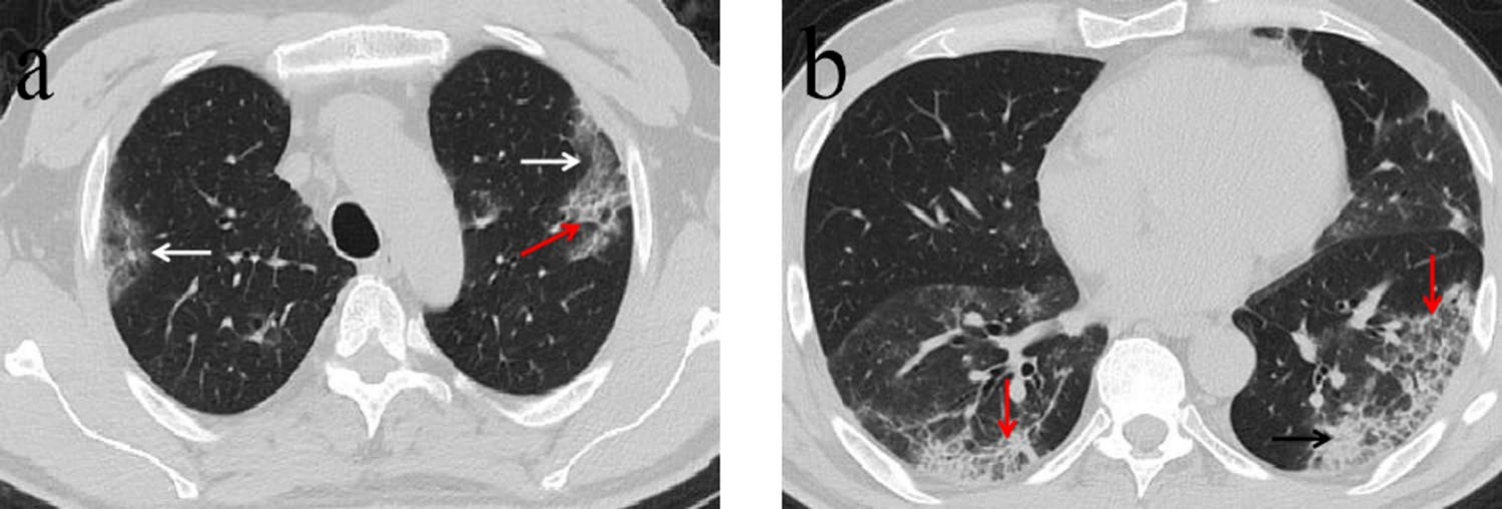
A 57-year-old man with a history of recent travel to Wuhan presented with fever for 10 days. (a,b) Non-contrast enhanced chest CT showed multiple peripheral patchy ground glass opacities (white arrows) and crazy-paving pattern (red arrow) in both lower lobes. CT scan also demonstrated consolidation in the left lower lobe (black arrow). CT involvement score was 11.
